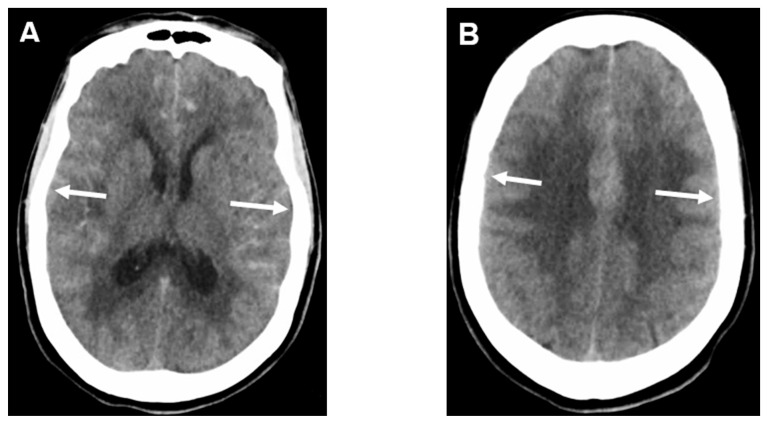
SEBES score calculation from non-contrast CT of the head. (A) Slice 1 with 2 points for bilateral effacement of sulci. (B) Slice 2 of the same CT with 2 points for bilateral sulcal effacement. The total SEBES score for this scan is 4.
